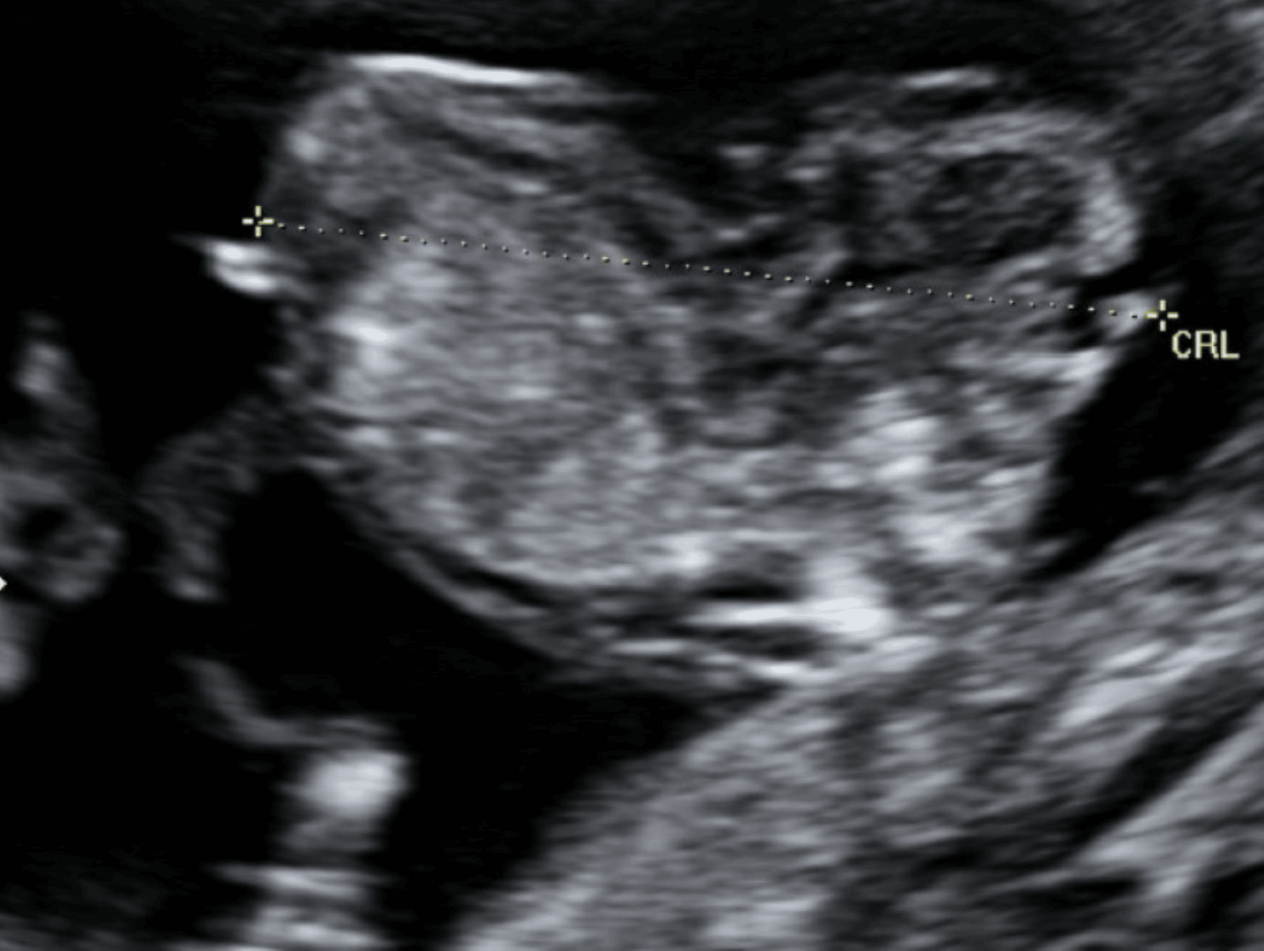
Ultrasound at 12 weeks and six days of gestation revealing the fetus with cervicothoracic hyperlordosis and absence of the neck
